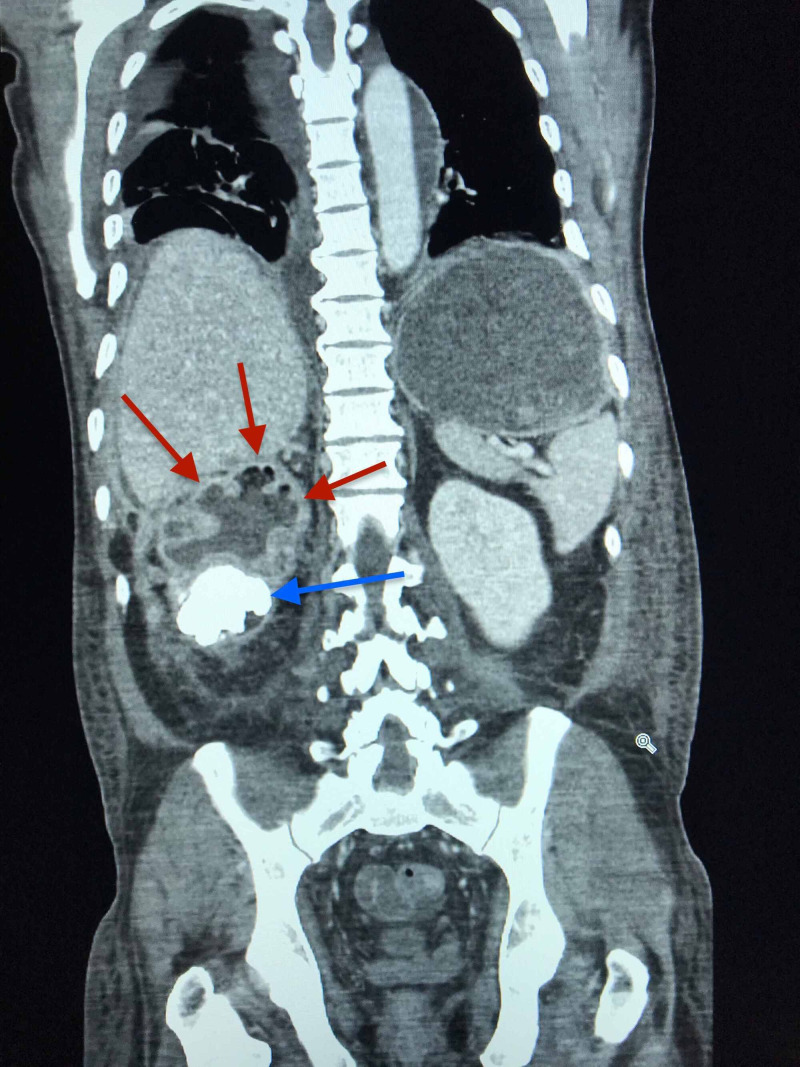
Right kidney visualized with staghorn calculi and dilated renal calyces on CT of abdomen, coronal plane Staghorn calculus is indicated by the blue arrow. Dilated renal calyces are indicated by the red arrows.
